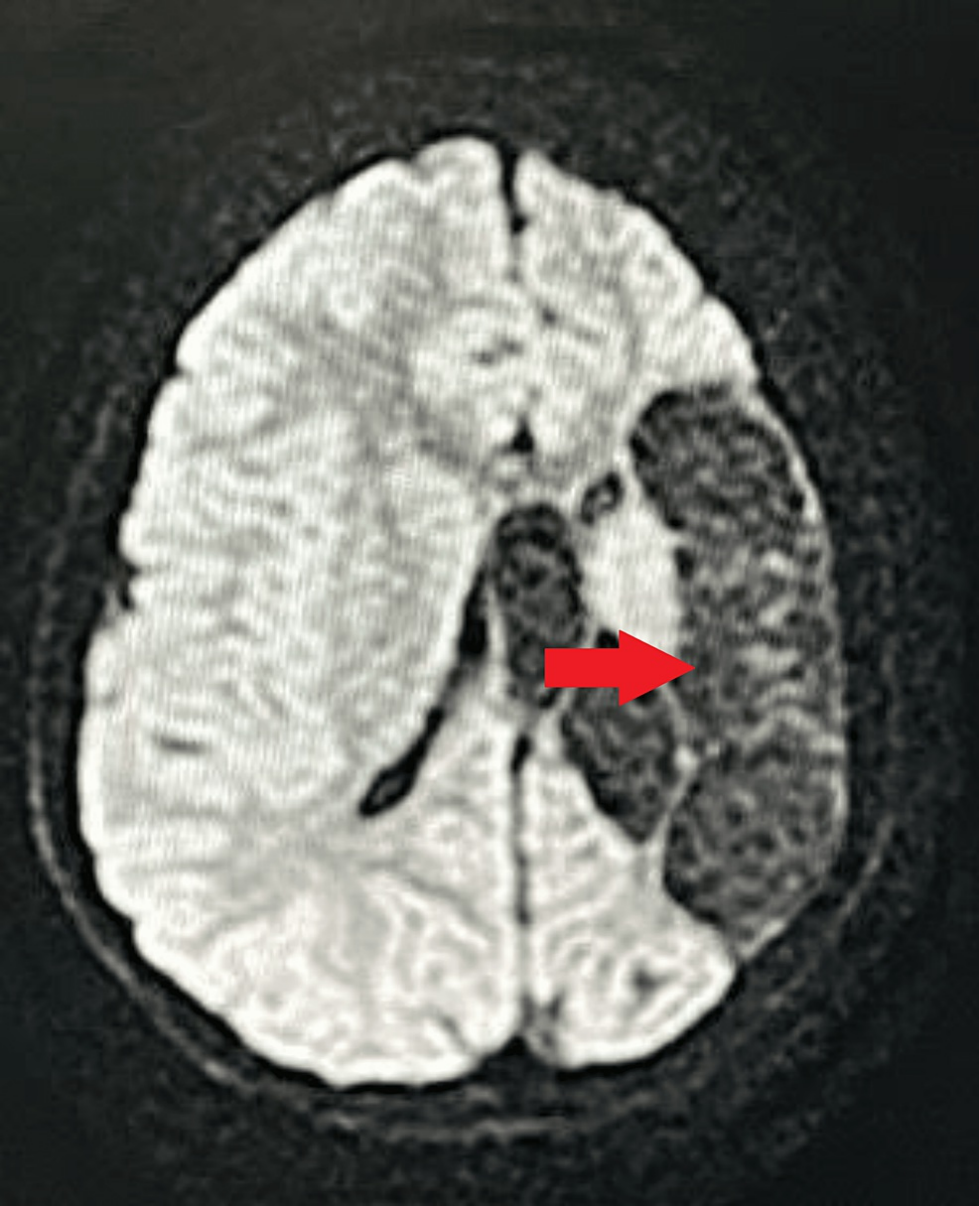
MRI brain axial DWI No evidence of restriction is noted at the frontoparietal lobe on the left side Red arrow: no evidence of restriction at the left frontoparietal lobe DWI: diffusion-weighted imaging; MRI: magnetic resonance imaging
